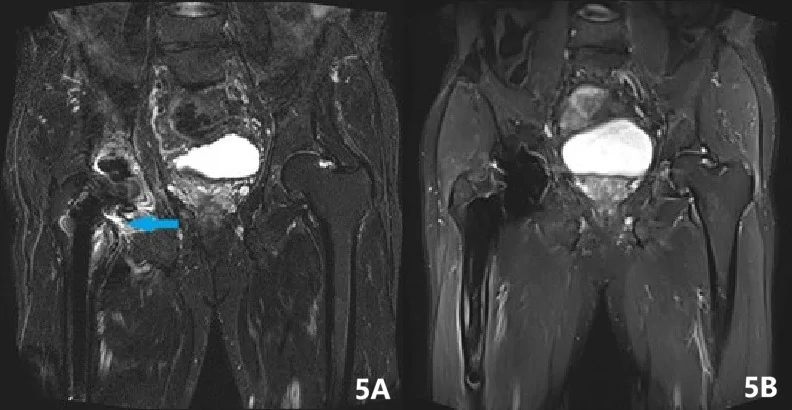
a) Preoperative metal artifact reduction sequence (MARS) MRI sequence image of the right hip debridement in a 70-year-old male, showing inflammatory oedema in the periprosthetic tissue as indicated by the blue arrow. b) Ten months after debridement surgery performed in the second‑stage procedure, pre‑reimplantation MARS‑MRI sequences were obtained.
